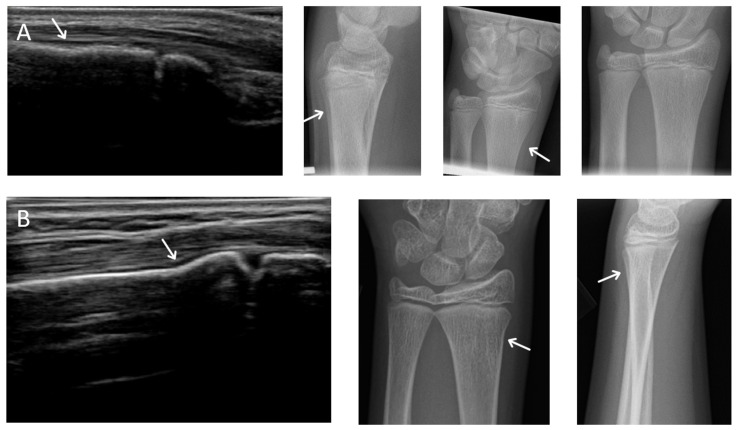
Possible missed fractures. (A,B) are dorsal longitudinal views of the distal radius of two possible X-ray false negatives. Note the excess angulation of the distal radius may represent torus fractures. The possible defect is observable on X-ray.
